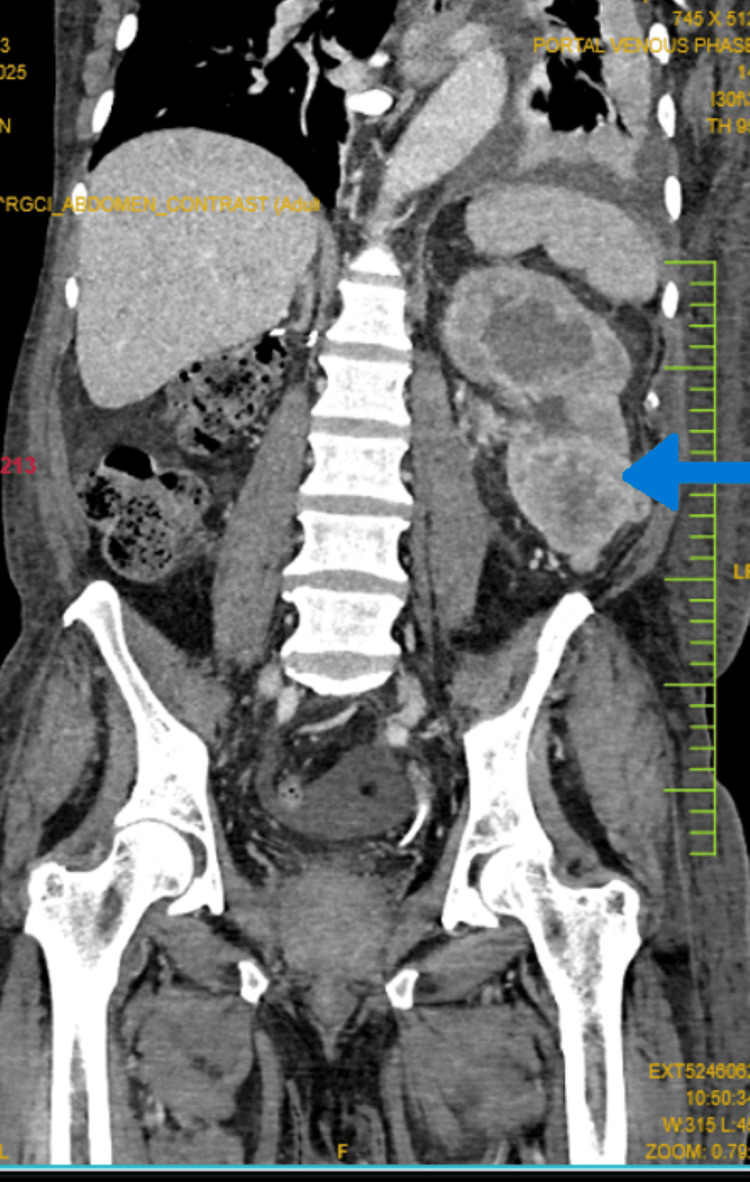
CT scan showing single large left kidney The left kidney appears diffusely bulky with a mildly enlarged exophytic growth at the lower pole (arrow). Post-right nephrectomy status is noted, with no definitive evidence of recurrent disease in the right renal fossa.
